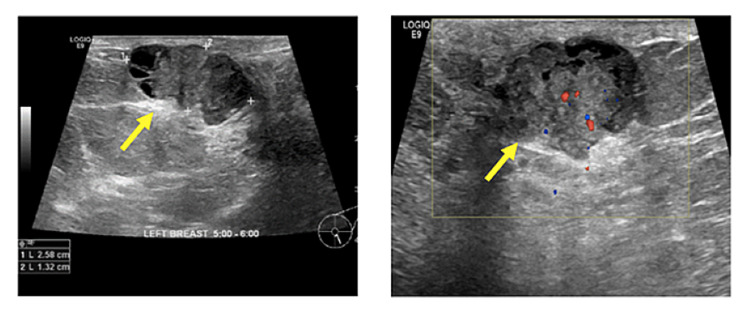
Pathology-proven papilloma with ultrasound findings of a solid lesion with a cystic component (yellow arrow).
